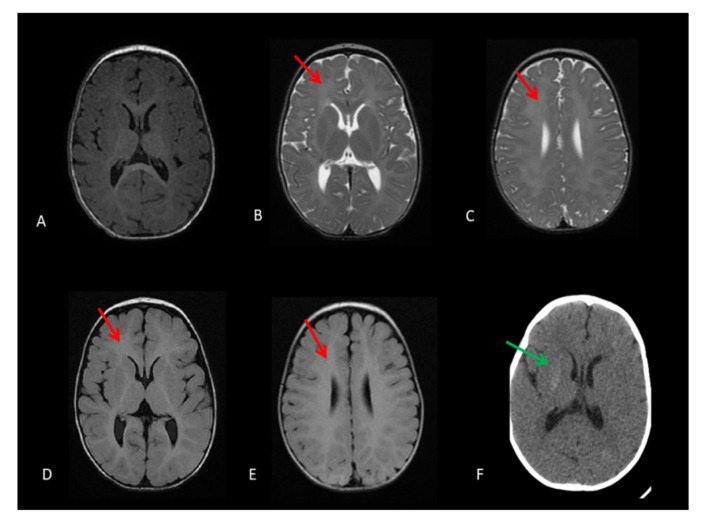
MRI images of patient CS10. (A) Axial T1-weighted image, (B,C) axial T2-weighted images, and (D,E) axial FLAIR-weighted images, showing isointensity of periventricular white matter on T1 and hyperintensity on T2. FLAIR suggestive of hypomyelinating leukodystrophy (red arrows). (F) CT scan shows lenticular calcifications (green arrow).
